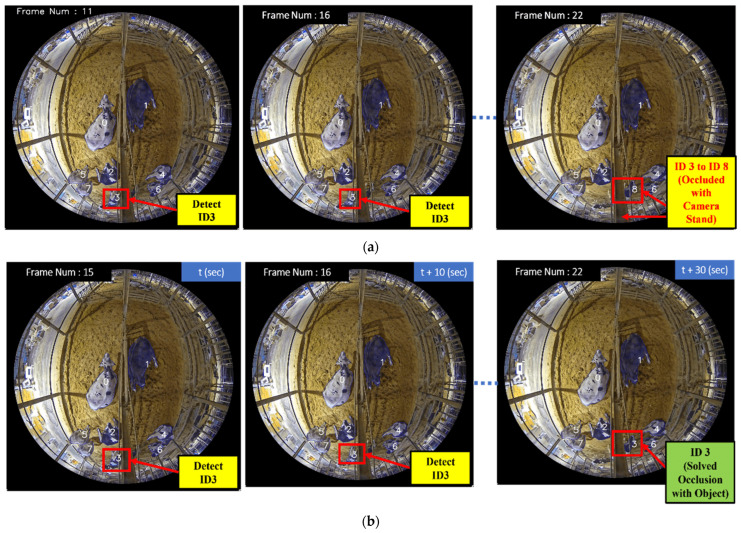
Comparison of occlusion with object case of using IOU and CTA: (a) IOU algorithm (which cannot solve the ID increment case); (b) CTA (which solved the ID increment case).
